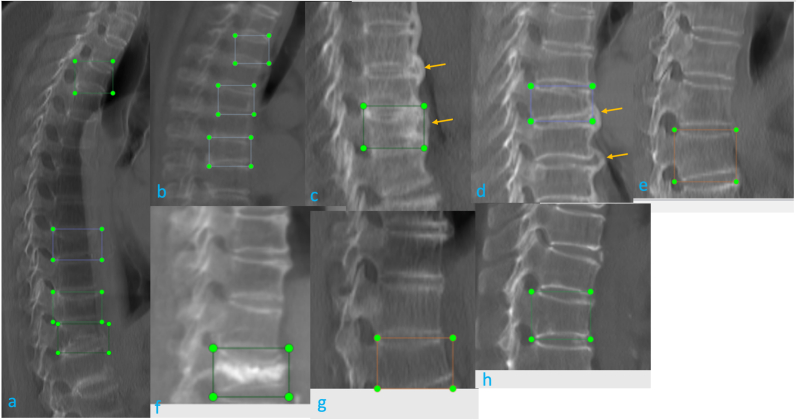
CSR CT images of 8 cases with 13 VF with false negative reading (labeled with green dotted box). A and B are associated with low signal of the vertebral, as the spine was off-center with scoliosis. C and B are associated with apparent formation of osteophytes (arrows). For E, F, G, and H, the VFs are close to the lower margin of field of view, without a full lower vertebra for reference.
